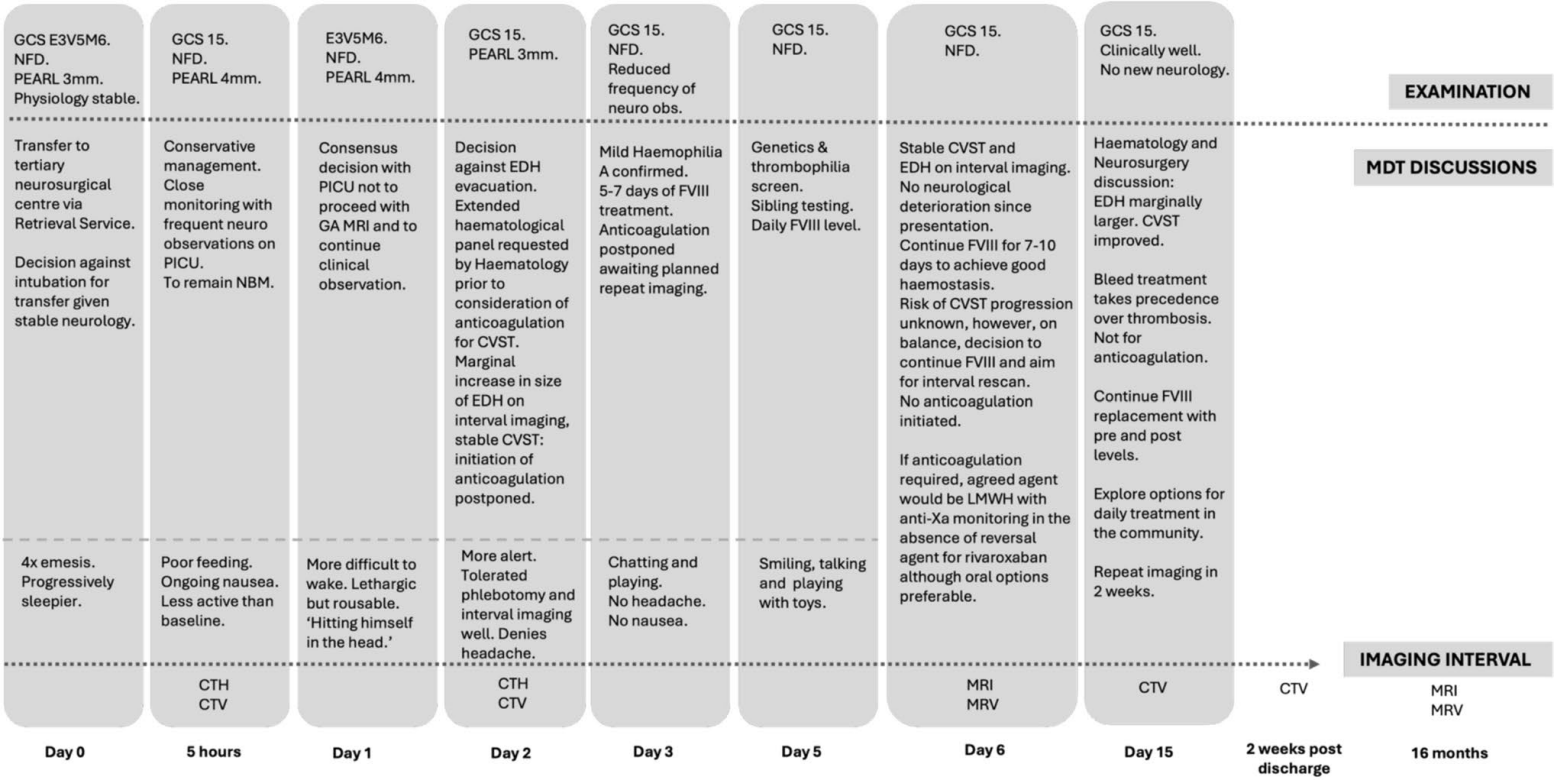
Timeline detailing the course of events from admission to outpatient follow-up. Details including evolution of symptomatology, serial examinations, MDT discussions and imaging intervals. LMWH, low molecular weight heparin; CTH, computed tomography head; CTV, computed tomography venogram; GCS, Glasgow Coma Scale; EDH, extradural haemorrhage; CVST, cerebral venous sinus thrombosis; MRI, magnetic resonance imaging; MRV, magnetic resonance venogram
